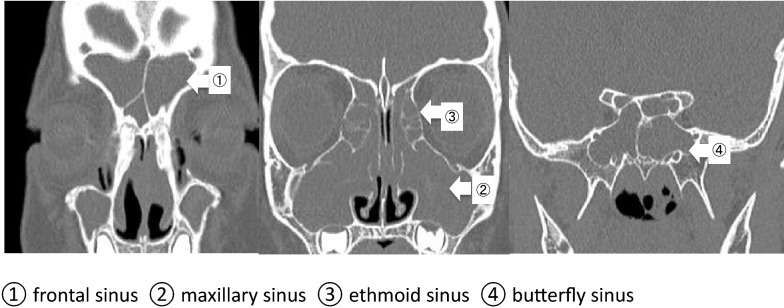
Paranasal computed tomography images before treatment. Pretreatment computed tomography at 8 years and 6 months of age shows paranasal sinusitis. The scans reveal secretory reservoirs in the frontal, maxillary, ethmoid, and butterfly paranasal sinuses
